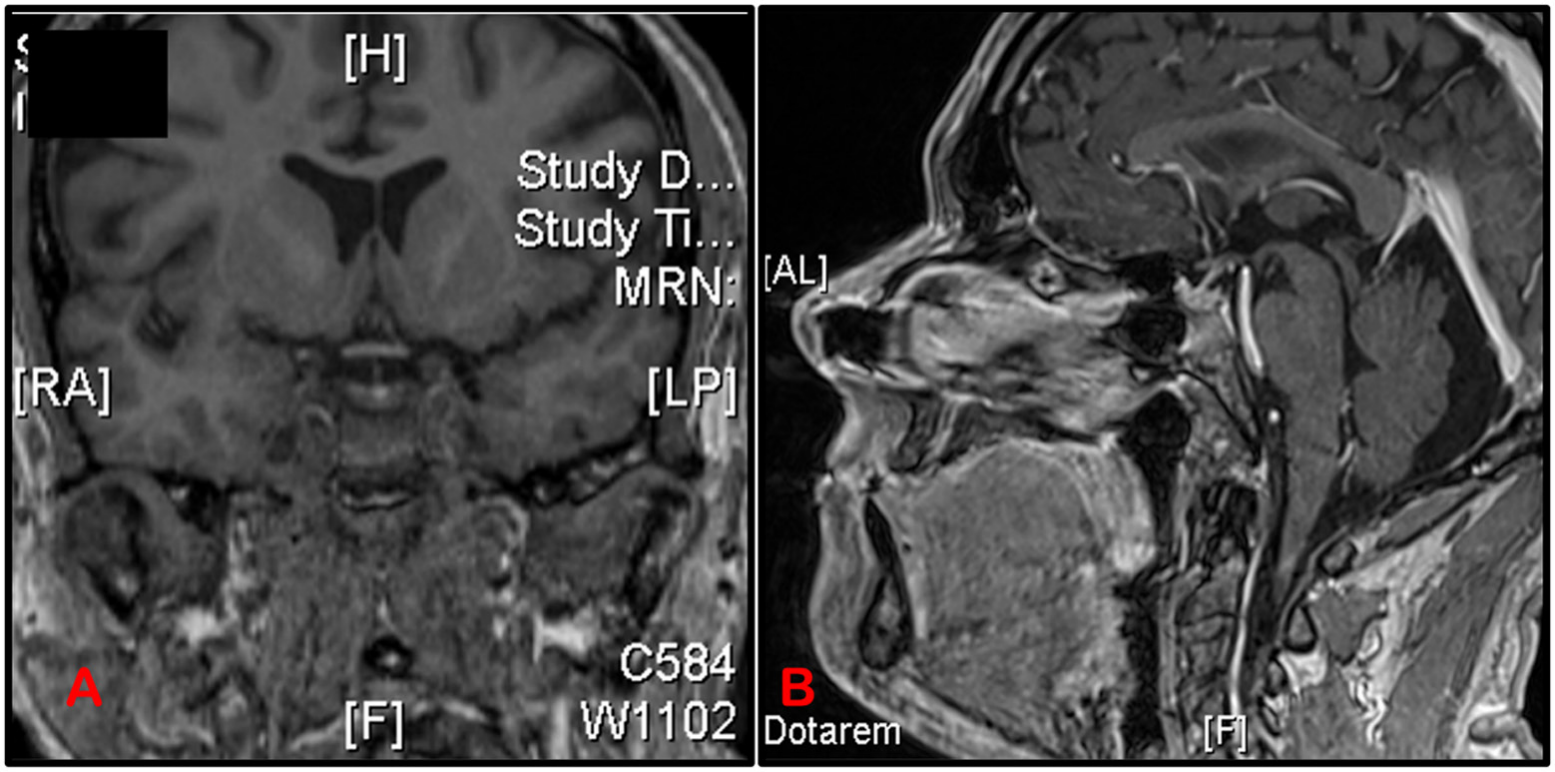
Coronal and sagittal T1 MRI sequences with contrast showing an unremarkable pituitary fossa, with no masses or pituitary enlargement to suggest hypophysitis. The pituitary stalk and hypophyseal region were also unremarkable. However, it is important to note that this MRI was performed 3 months following the initial SST and early inflammatory changes might have resolved by the time of the scan.
